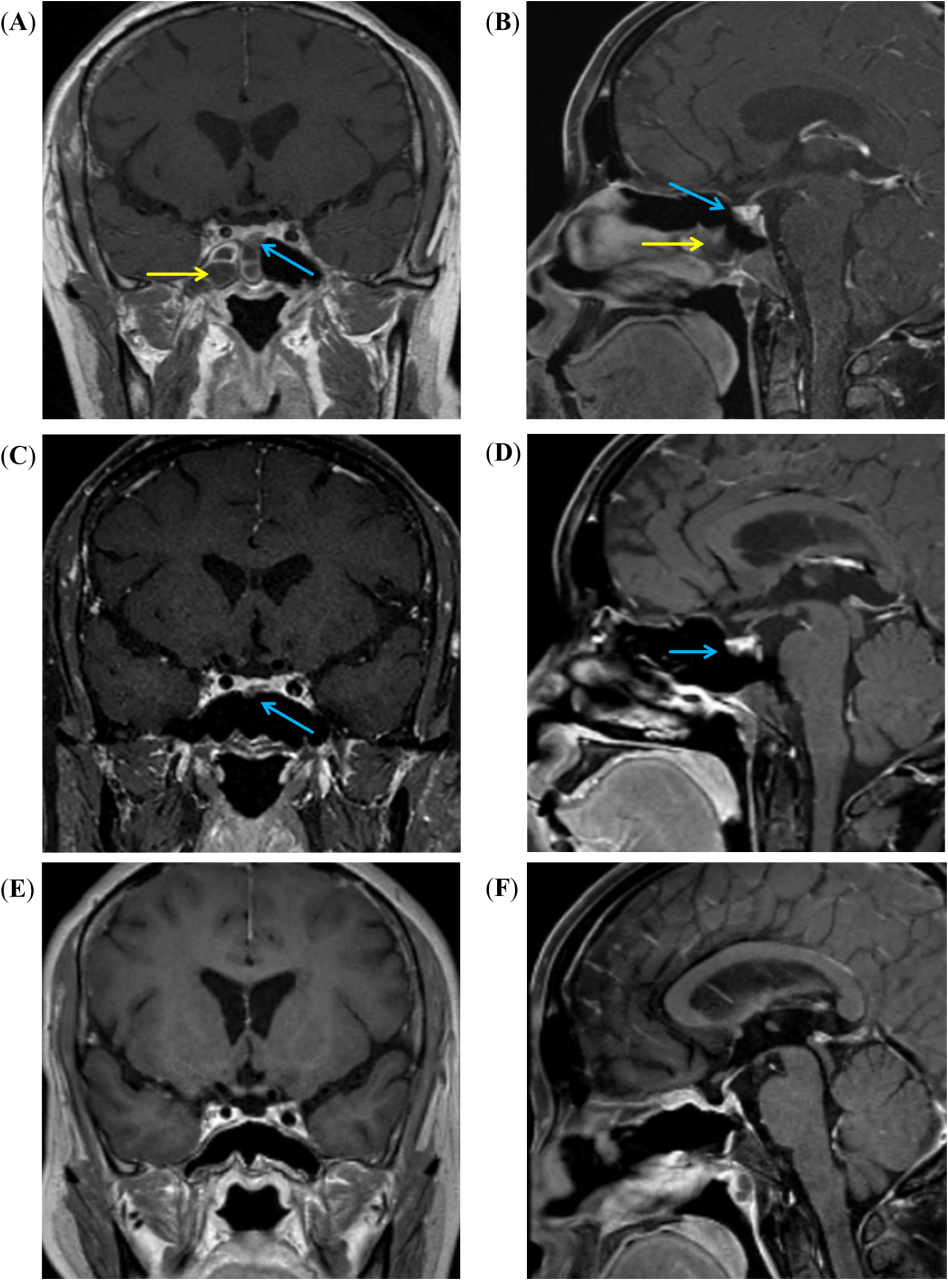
MRI images of the sellar region. (A, B) Coronal and sagittal T1-weighted enhanced MRI images show right sphenoid sinusitis (yellow arrow), and a low-signal intensity mass (blue arrow) is noted in the anterior-middle portion of the pituitary gland. (C, D) After 3 months of treatment with voriconazole, the right sphenoid sinusitis has resolved, but the mass (blue arrow) remains visible. (E, F) After the surgery, the pituitary tumor was resolved.
